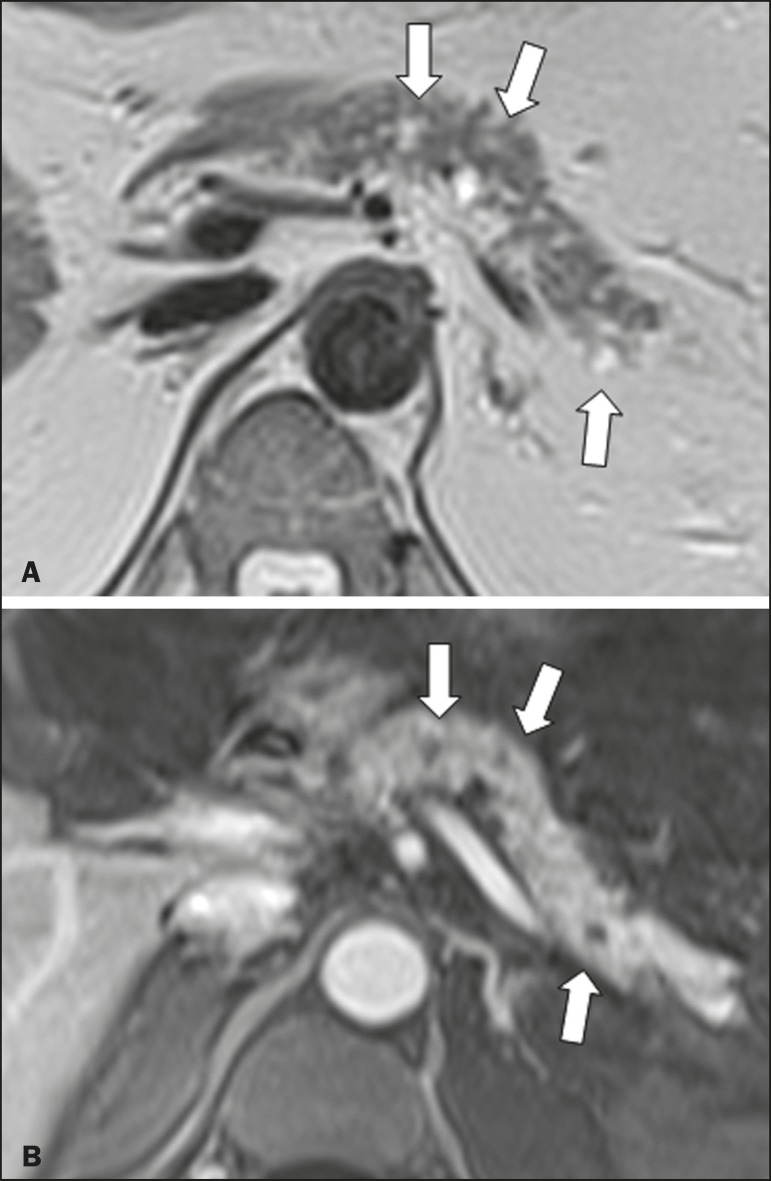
MRI scans. T2-weighted sequence (A) and contrast-enhanced
